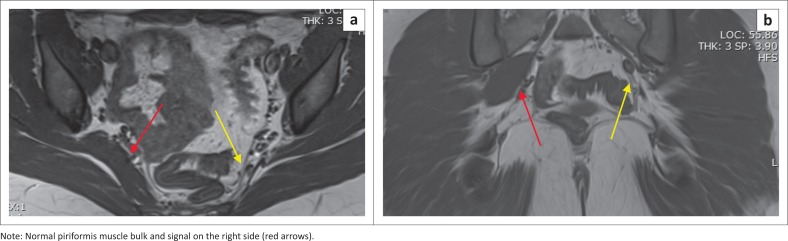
A 28-year-old woman who presented with low back pain for a month without neurological symptoms: (a) axial and (b) coronal T1-weighted images of the pelvis show congenital absence of the left piriformis muscle (yellow arrows point to the expected location).
